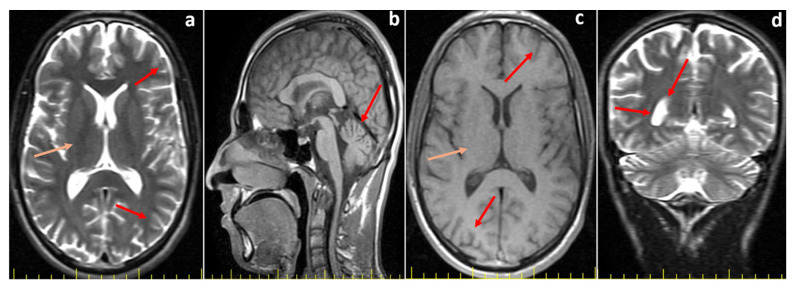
Cranial MR images of the index patient (Proband 1, P1) at 20 years of age. Cranial MR images of the index patient (Proband 1, P1) at 20 years of age. (a) T2-weighted axial image shows normal cerebral sulci (red arrows) and basal ganglia (orange arrow). (b) T1-weighted sagittal image reveals mild cerebellar atrophy (red arrow). (c) T1-weighted axial image shows normal cerebral sulci (red arrows) and basal ganglia (orange arrow). (d) T2-weighted coronal section demonstrates normal ventricular volume (red arrows).
